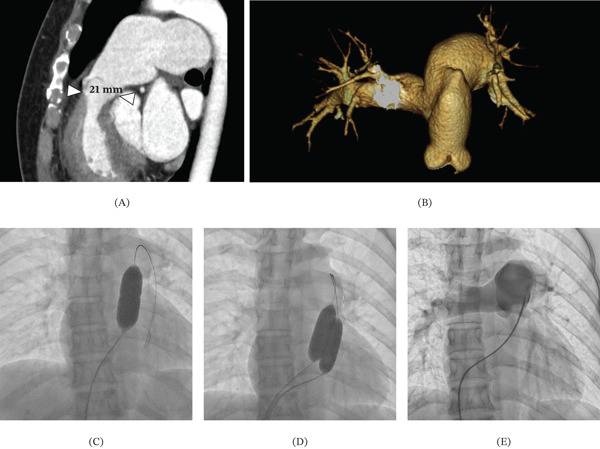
Preprocedural imaging and interventional procedure. (A) Contrast‐enhanced computed tomography acquired during systole demonstrated a 21‐mm pulmonary valve annulus (white arrowheads), without calcification or evidence of supravalvular or subvalvular stenosis or focal narrowing of the right ventricular outflow tract. (B) Post‐stenotic dilatation of the main pulmonary artery. (C) Initial balloon pulmonary valvuloplasty using a single 22‐mm balloon (2 atm), which resulted in a residual pressure gradient. (D) Subsequent double‐balloon dilation with two 16‐mm balloons inflated simultaneously (2 atm each), achieving more effective valvular expansion and a substantial reduction in the pressure gradient. (E) Final pulmonary angiography showing only mild pulmonary regurgitation.
